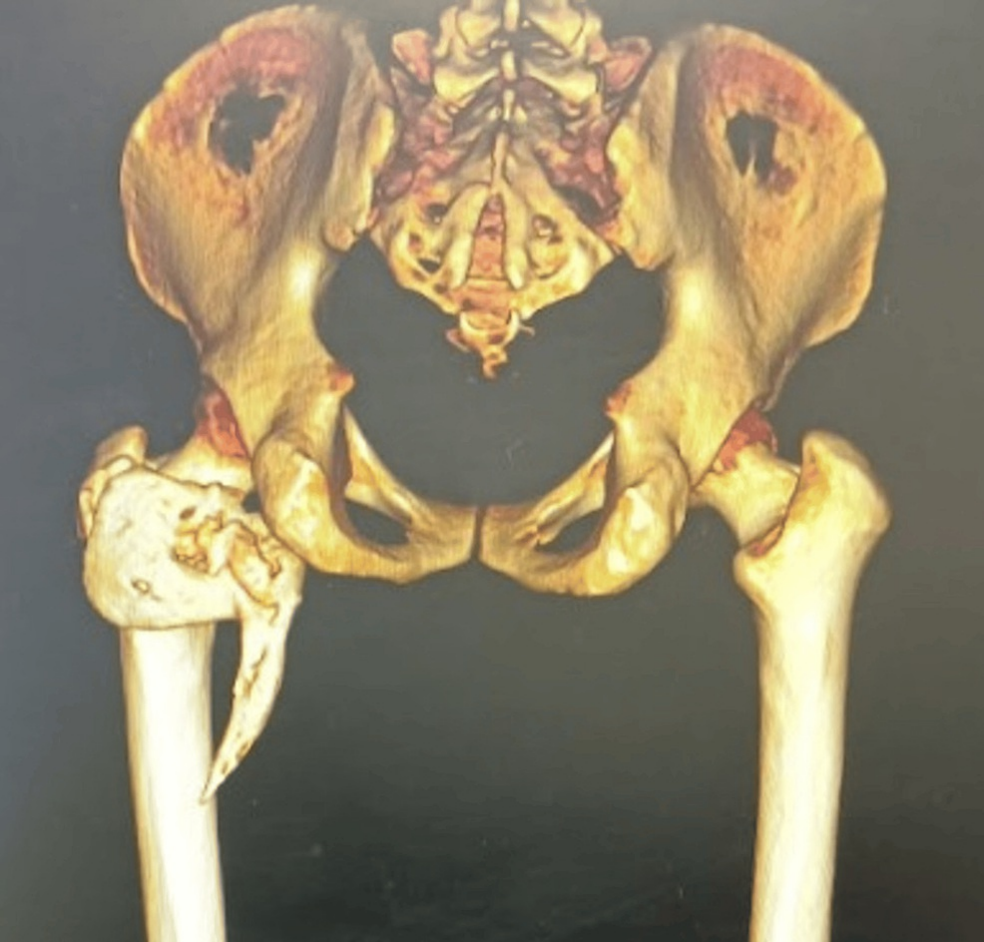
Pre-operative CT scan showing an irregular bony mass extending from gluteal region to subtrochanteric area of femur
